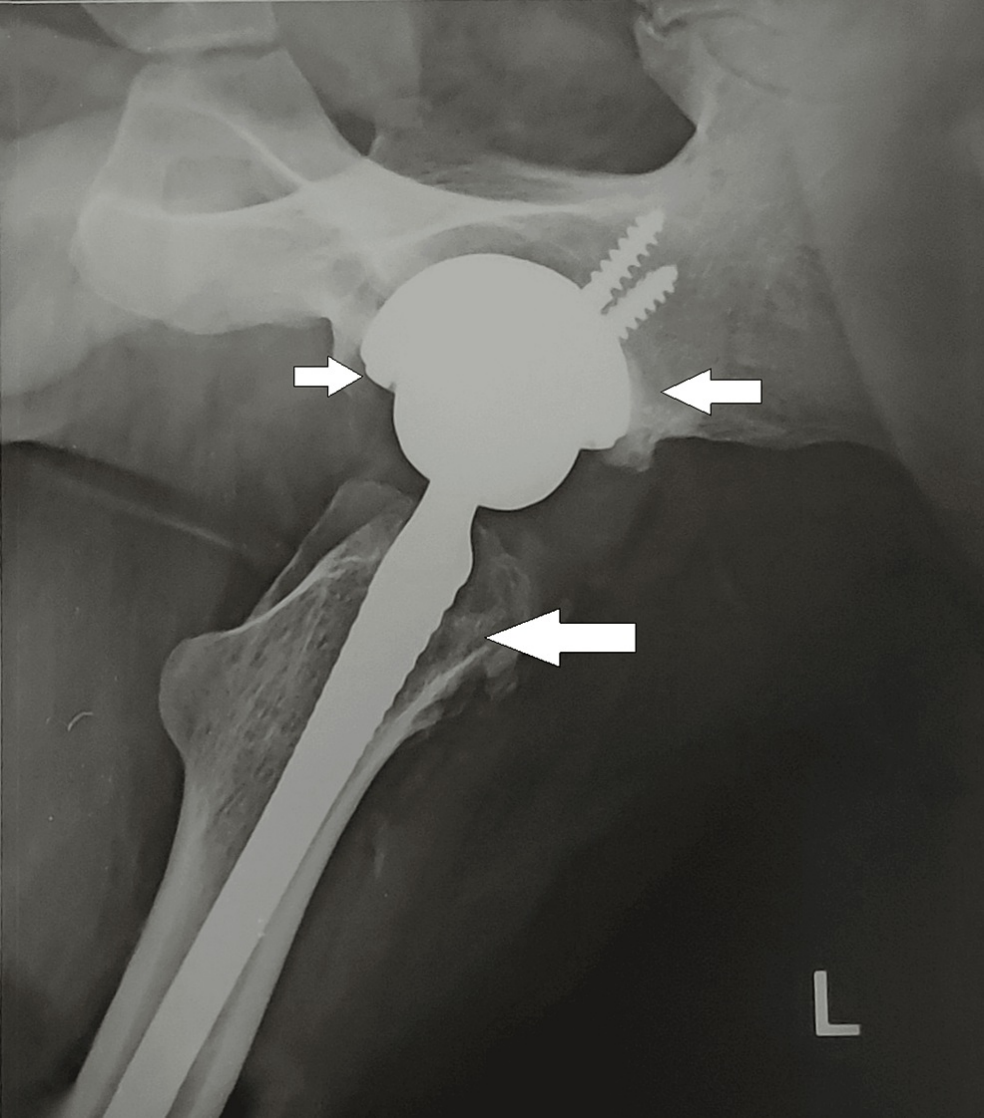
Lateral view of an X-ray of the pelvis showing total hip replacement on the left side.
